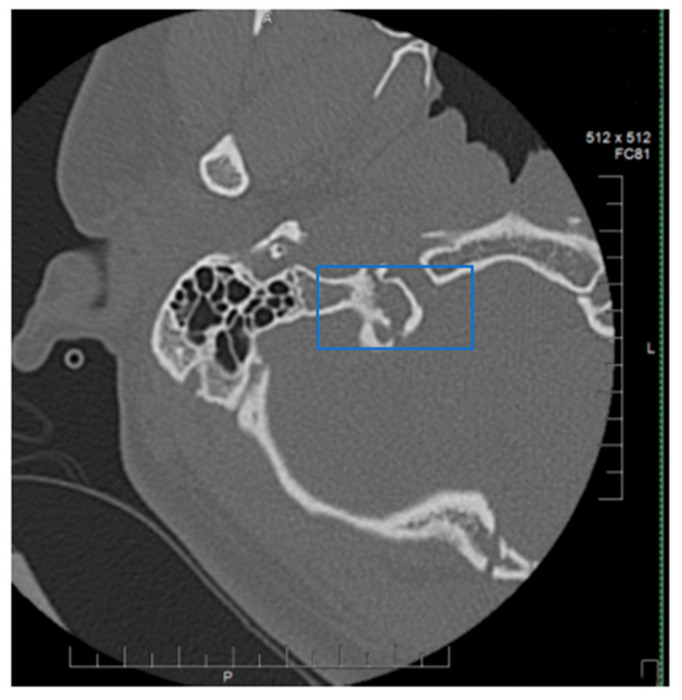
CT temporal bone. Highlighted blue box indicates junction of Jugular foramen and Hypoglossal Canal.
